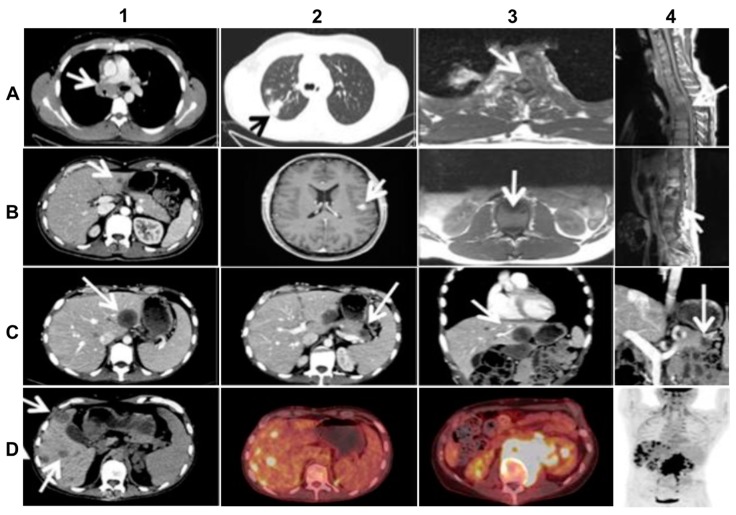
Imaging of the advanced (stage IV) *ALK*-rearranged NSCLC at diagnosis and at relapse after sequential treatment with Crizotinib, Alectinib, and re-challenge with Alectinib (*relevant changes indicated by arrows*). (A) top line from left to right: diagnostic CT (A1,A2) and MR (A3,A4) scans at baseline showing metastasized mediastinal lymph nodes compressing the vena cava superior (A1), the primary lung tumor in the right upper lobe (A2), transversal (A3) and sagittal (A4) images of vertebral metastases with spinal cord affection; (B) second line from left to right: CT (B1,B2) and MR (B3,B4) scans at progression after 10 months of Crizotinib treatment, showing liver metastasis (B1), the largest brain metastasis (B2), transversal (B3) and sagittal (B4) images of new vertebral metastases affecting the spinal cord; (C) third line from left to right: CT scans (C1–4) after 3 months of Alectinib treatment, exhibiting mixed response including new hepatic (C1,C3) and pancreatic (C2,C4) metastases on transversal and frontal planes; (D) last line from left to right: CT-(D1), PET/CT- (D2, D3), and upper part of total-body PET-scan (D4) at progression after re-challenge with Alectinib, showing multiple new hepatic metastases (D1,D2,D4) and a large retroperitoneal conglomerate of metastasized lymph nodes involving the left kidney (D3, D4).
